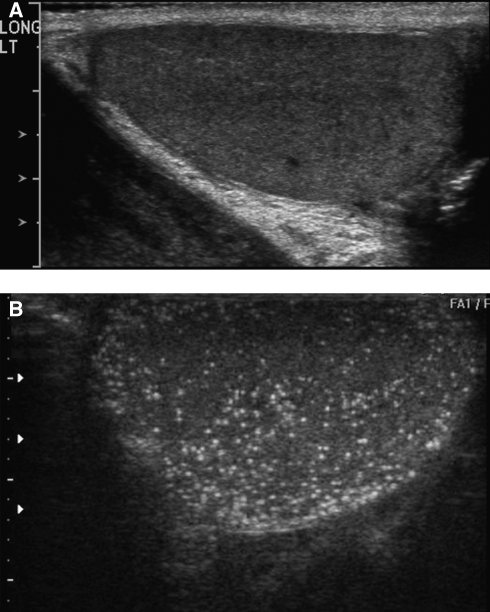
Testicular ultrasounds depicting (A) normal exam and (B) extensive microlithiasis.
